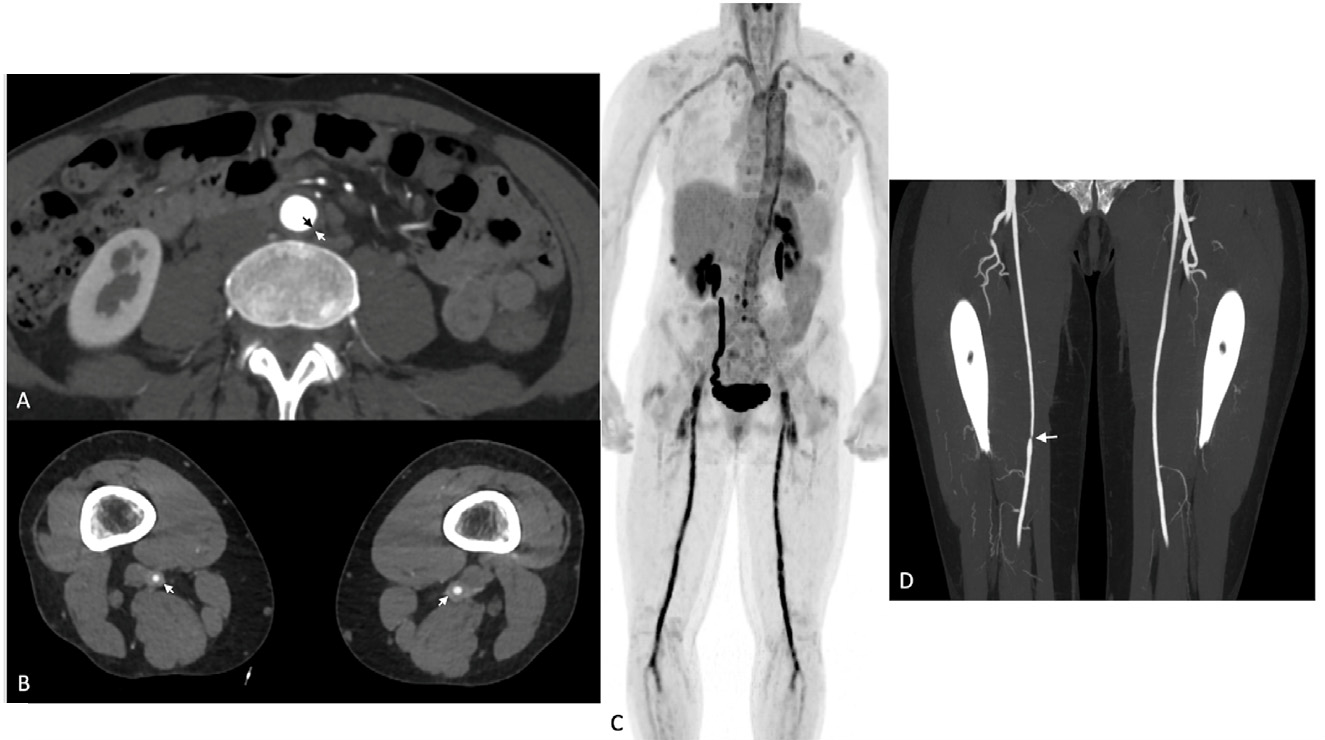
A 59-year-old female presented with claudication. Contrast-enhanced CTA of the abdomen/pelvis (Panel A) and lower extremities (Panel B) revealed circumferential wall thickening of the aorta and lower extremity arteries (arrows). Subsequent FDG-PET/CT imaging (Panel C) demonstrated moderate-to-intense FDG uptake in the corresponding vessels. A cranial ultrasound demonstrated the halo sign (not shown), and a subsequent temporal artery biopsy confirmed the diagnosis of GCA. Follow-up CTA after immunosuppressive therapy (Panel D) showed resolution of the wall thickening, but with severe stenosis of the distal superficial femoral artery (arrow). CTA, computed tomography angiography; FDG-PET/CT, [18F]-fluoro-2-deoxy-d-glucose positron emission tomography/computed tomography; GCA, giant cell arteritis.
